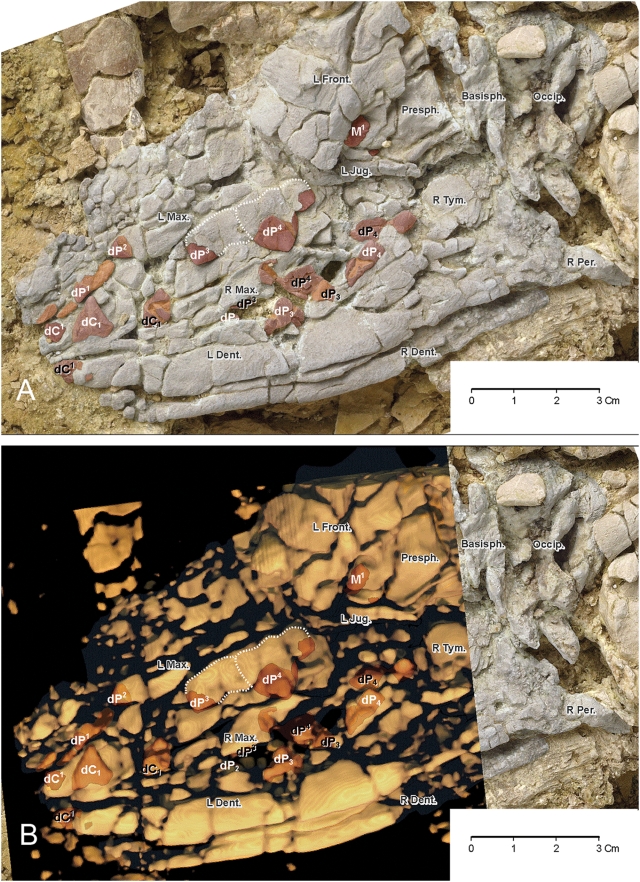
Fetal skull of *Maiacetus inuus* (GSP-UM 3475b). (A)–Photograph showing bones (shaded white) and teeth (shaded brown) in lateral view. (B)–CT image overlain on tooth-bearing part of fetal skull in lateral veiw. *Abbreviations*: left frontal (*L. Front.*), left jugal (*L Jug.*), occipital (*Occip*.) with left and right condyles flanking the foramen magnum, and left and right dentaries (*L dent.*, *R dent.*). Teeth are shaded brown, with darker brown representing enamel, and lighter brown representing roots and exposed dentine. White and black labels identify teeth of the left and right sides, respectively. Dotted lines trace outlines of fully formed crowns of left dP^3^ and dP^4^. These crowns are visible on the surface (A) where thin bone of the maxilla is pressed over more rigid underlying crowns, and as denser masses in the CT scan (B). Remaining teeth are identified by size and position relative to dP^3^ and dP^4^. Note presence of the developing crown of permanent left M^1^ posterodorsal to the crown of left dP^4^ (dorsal to the left jugal and posterior to the left frontal). Partial crown of right M^1^ (unlabeled) is visible just below and posterior to left M^1^.
